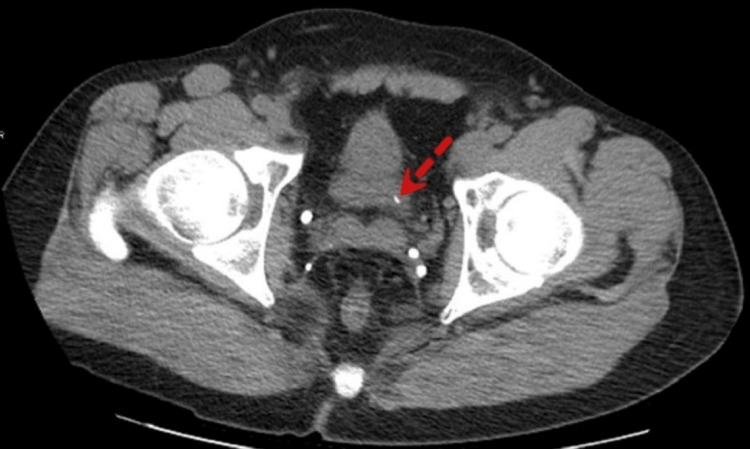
A renal stone (red dashed arrow) was detected in the left ureterovesical junction (computed tomography without contrast, axial view).
